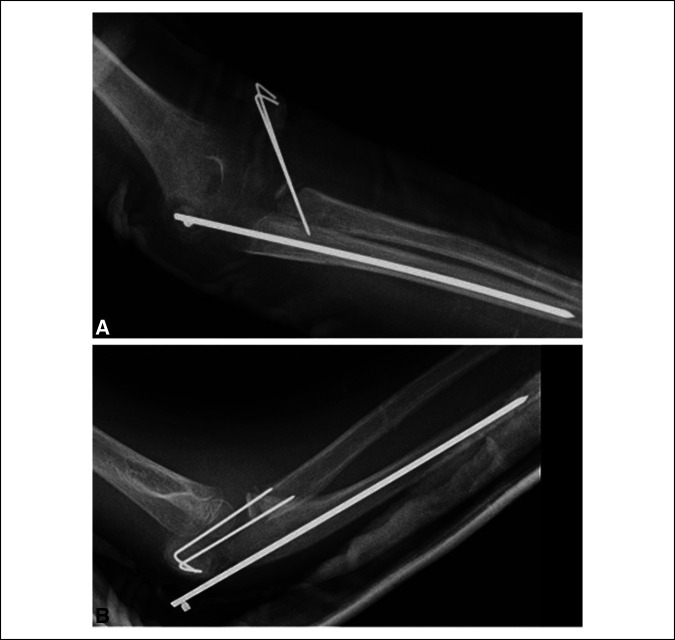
Radiographs showing two views of the left elbow before pin removal.
